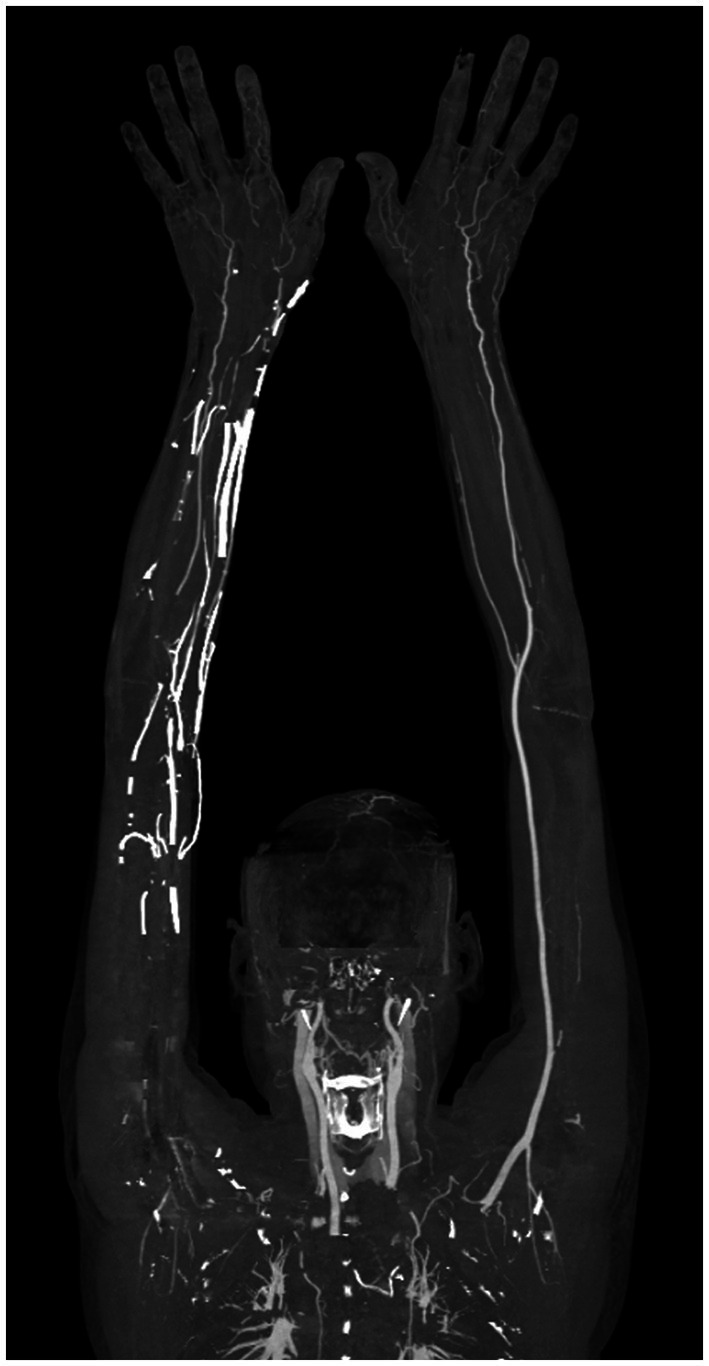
Computed tomography angiogram maximum-intensity projection of both upper limbs. This available archived image provided an overview of the upper-extremity arterial circulation. Although a discrete intraluminal thrombus or sharply defined contrast cutoff could not be optimally visualized on this single reconstruction, the image is concordant with the radiology-reported cessation of radial artery flow at the wrist bilaterally.
